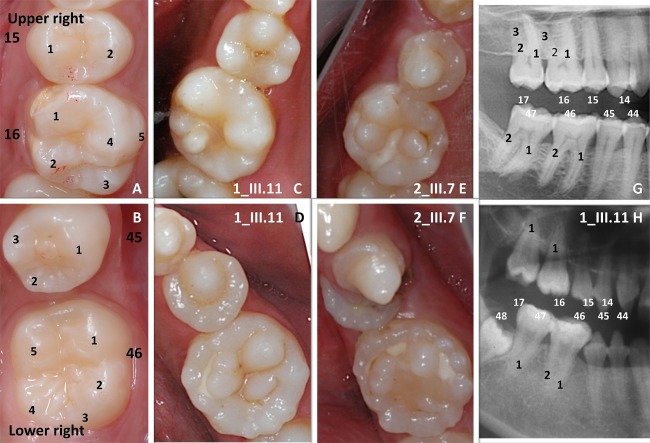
A striking multiple cusps single root phenotype. Normal crown morphology (A,B) of the right upper second premolar (15) and first permanent molar (16) and of the right lower premolar (45) and first permanent molar (46) could be compared to the multicusp pattern phenotype clearly visible both in the upper (C 1_III.11, E 2_III.7) and lower (D 1_III.11, F 2_III.7) jaws. On the close up of the right premolar-molar area on panoramic radiographs (G control, H 1_III.11 affected) molars present a single root (1) pattern. Only the first lower permanent molar (46) shows a more apical root furcation leading to the taurodontic appearance of the tooth.
